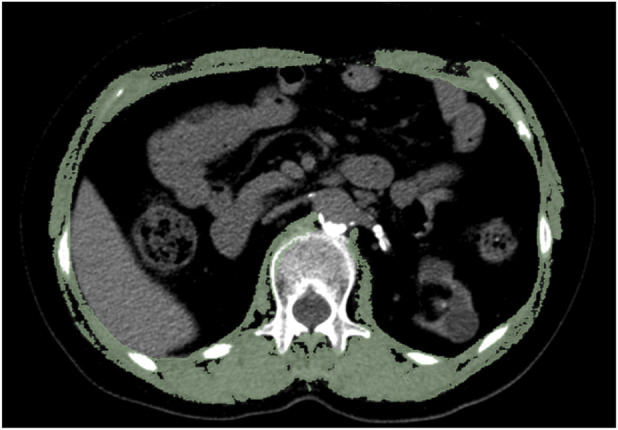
CT image showing skeletal muscle at the L1 level. The green section shows skeletal muscle at the L1 level, and the threshold ranges from −29 to +150 HU.
